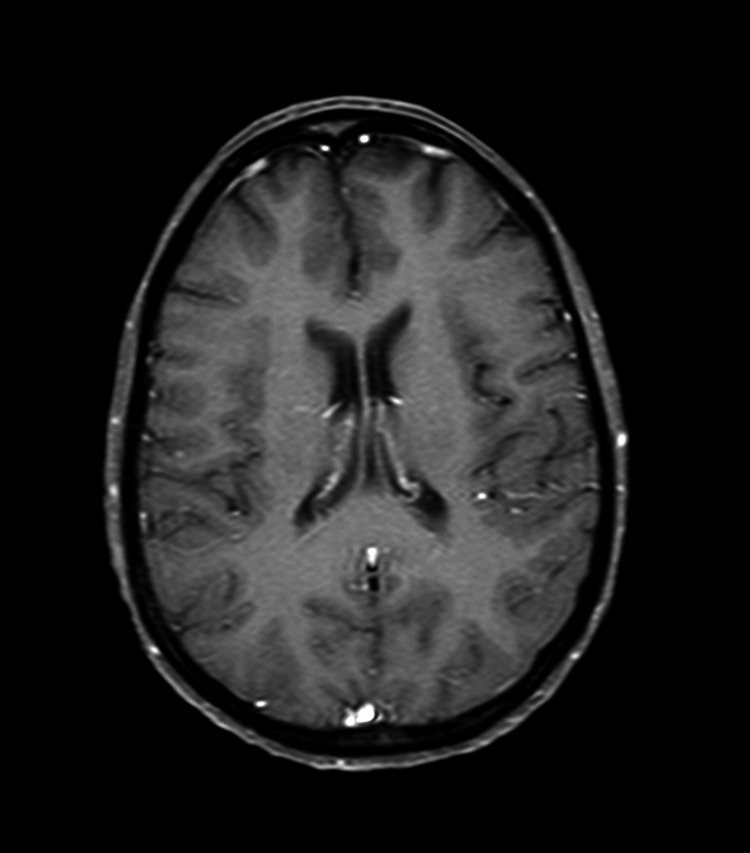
T1-weighted axial brain magnetic resonance image with contrast on April 25, 2019
